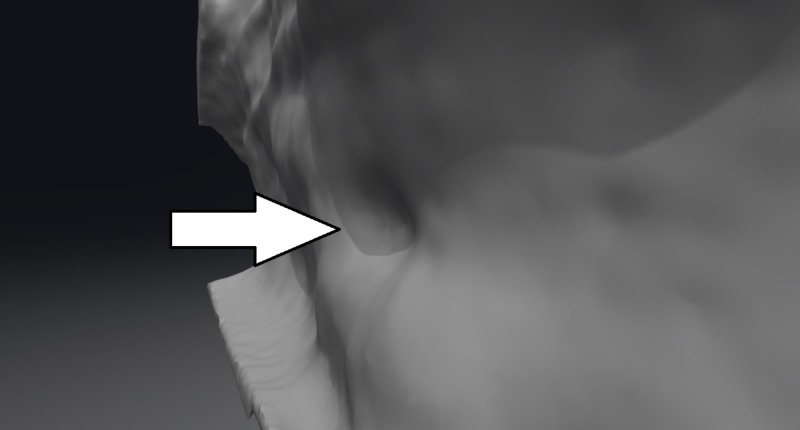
3D reconstruction of the nasal septum from a CT scan of the patient from the previous figure with the vomeronasal organ visible (arrow) 3D: three dimensional; CT: computer tomography
